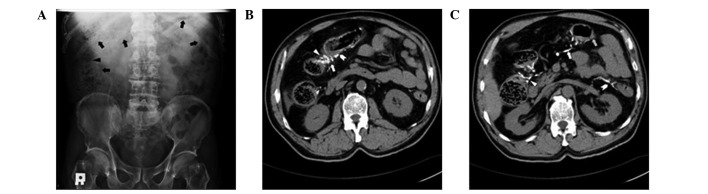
(A) Abdominal radiograph revealed fecal material in the right colon (arrowhead) with thread-like radiopaque densities representing vascular calcifications over the ascending and transverse colon (arrows). (B) CT revealed numerous serpentine calcifications (arrow) and stenosis (arrowhead) of the hepatic flexure of the colon with wall thickening (arrow tail). (C) CT also showed serpentine calcifications of the superior mesenteric vein and its tributaries of the ascending and transverse colon (arrowhead and arrow). Marked calcification of the inferior mesenteric vein and its tributaries of the descending colon was noted (arrow tail). In addition, wall thickening of the ascending, transverse and proximal descending colon, associated with poor contrast enhancement, was observed (arrow with oblique lines). CT, computed tomography.
